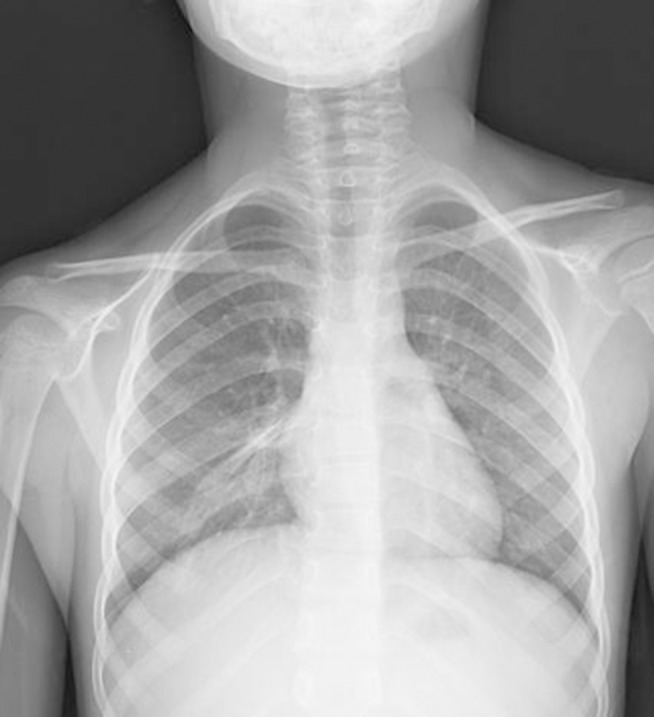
Chest X-ray; right perihilar hyperlucent appearance
